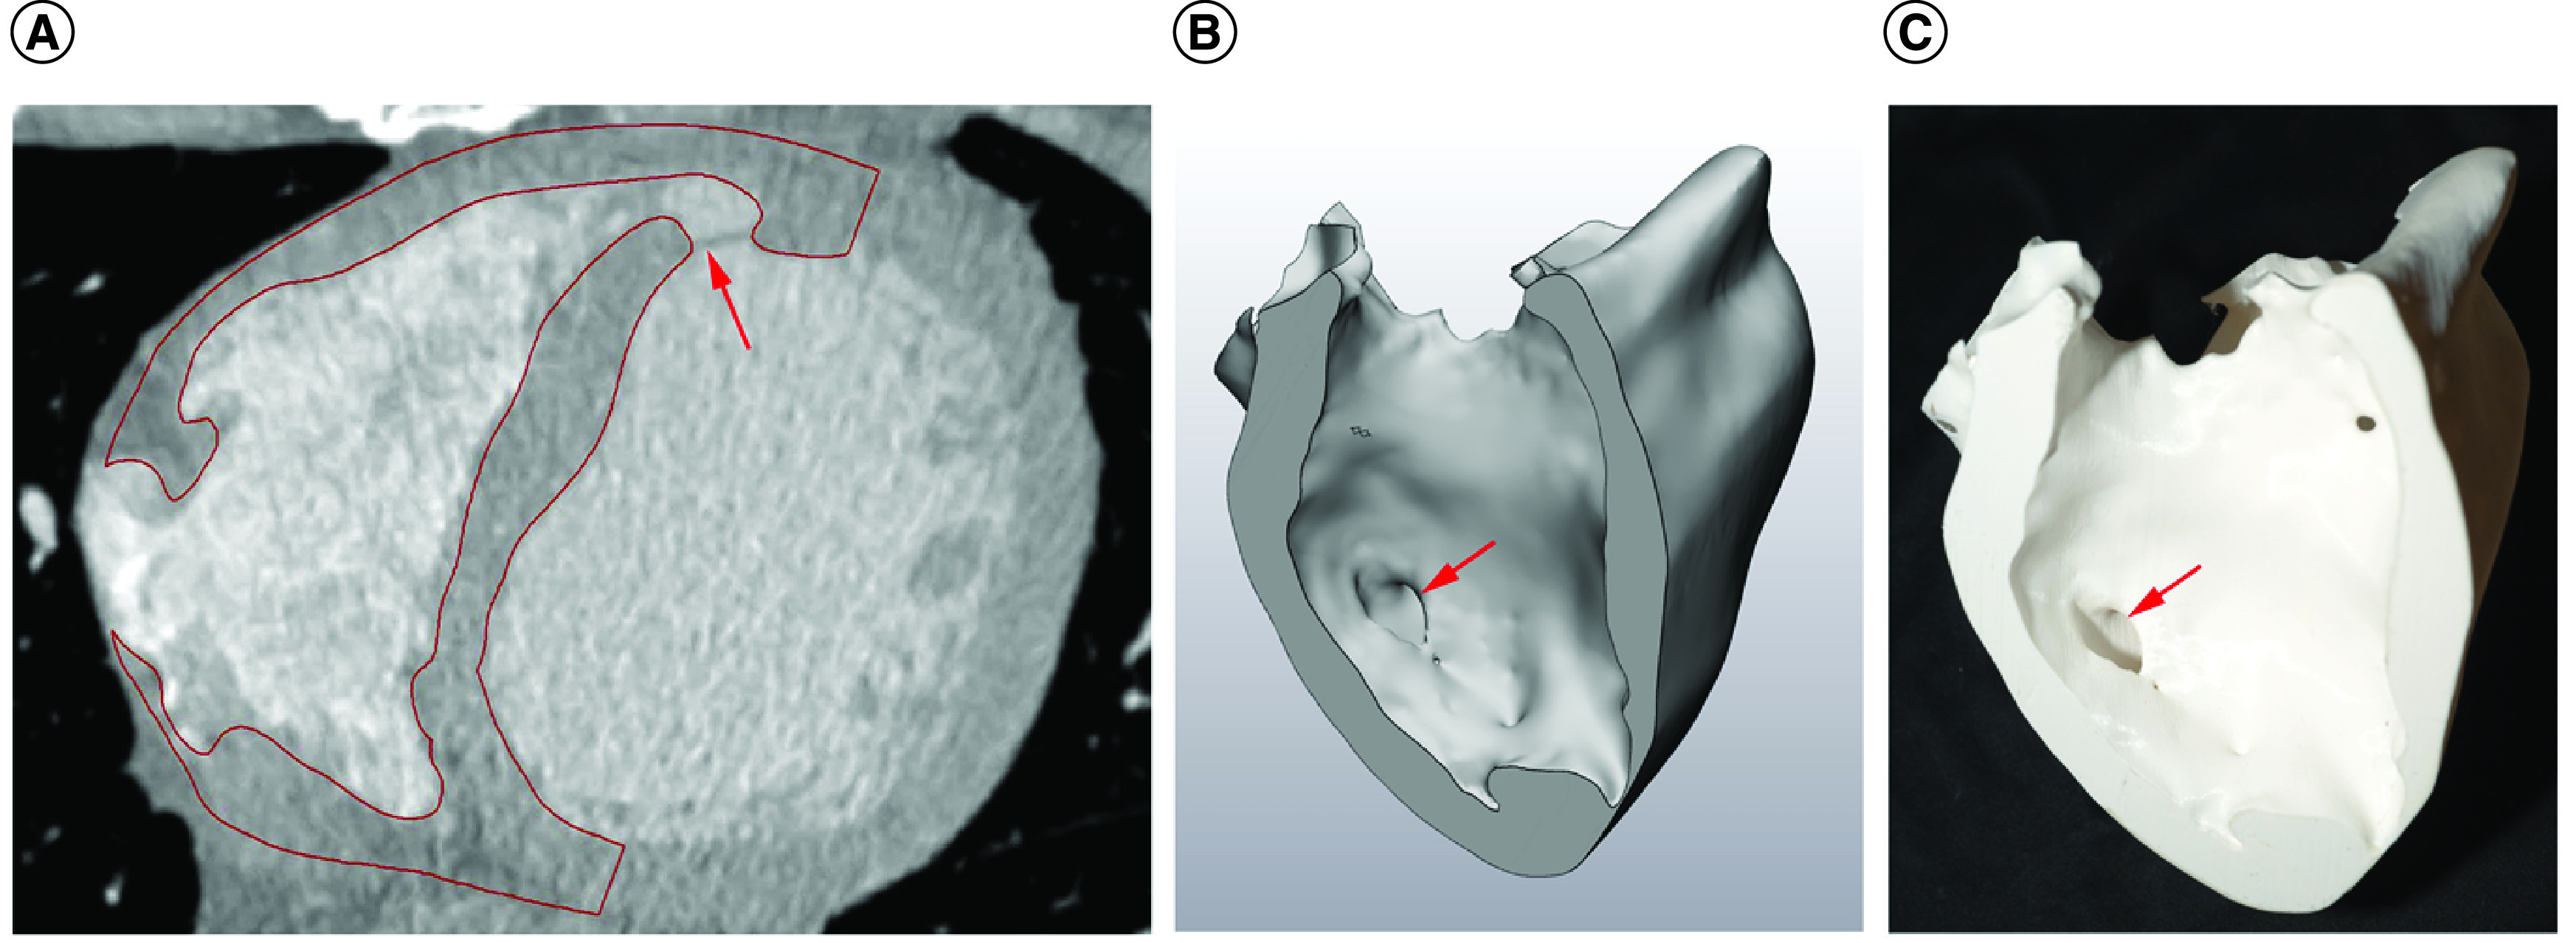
Segmentation of computed tomography to produce the 3D models. (A) The computed tomography image is segmented and a mask produced, which is converted to (B) a 3D volume. (C) This is, in turn, converted into a stereolithography file suitable for printing a final model. The ventricular septal defect is indicated by the arrow.
